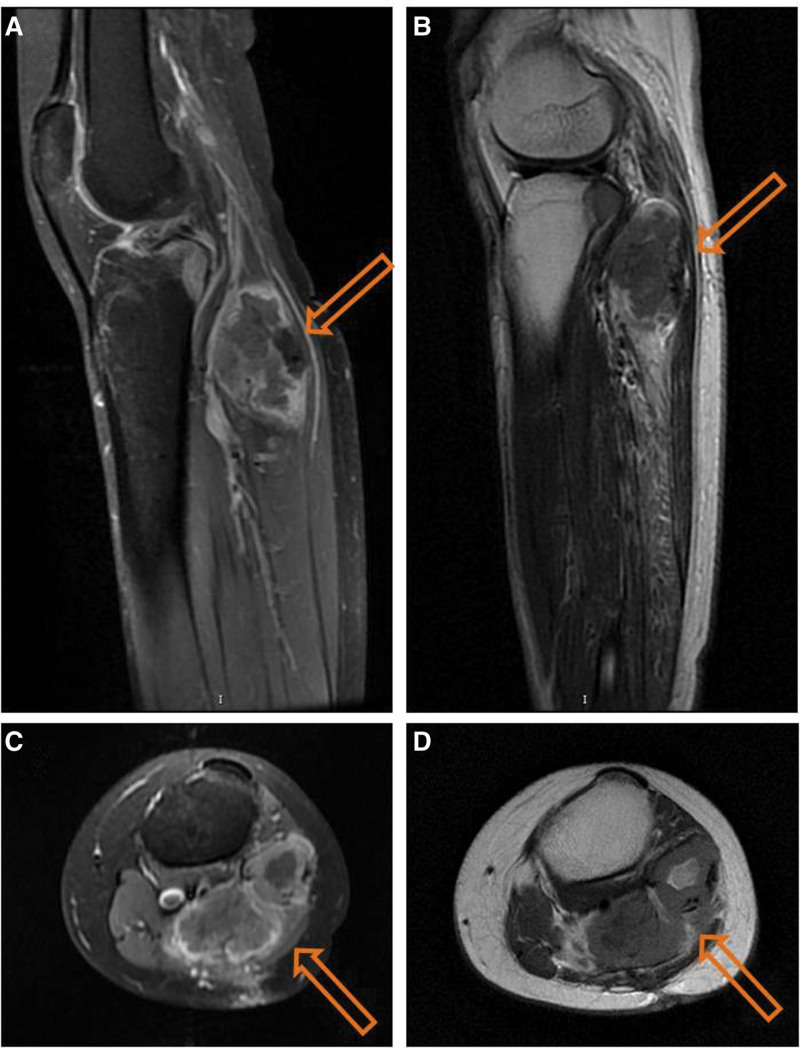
MRI of left leg mass. Sagittal image: (A) before targeted therapy (58 mm × 39 mm); (B) after 4 cycles of anlotinib hydrochloride therapy (53 mm × 35 mm). Axial image: (C) before targeted therapy (34 mm × 65 mm); (D) after 4 cycles of anlotinib hydrochloride (30 mm × 59 mm) (response evaluation criteria in solid tumors as stable disease).
